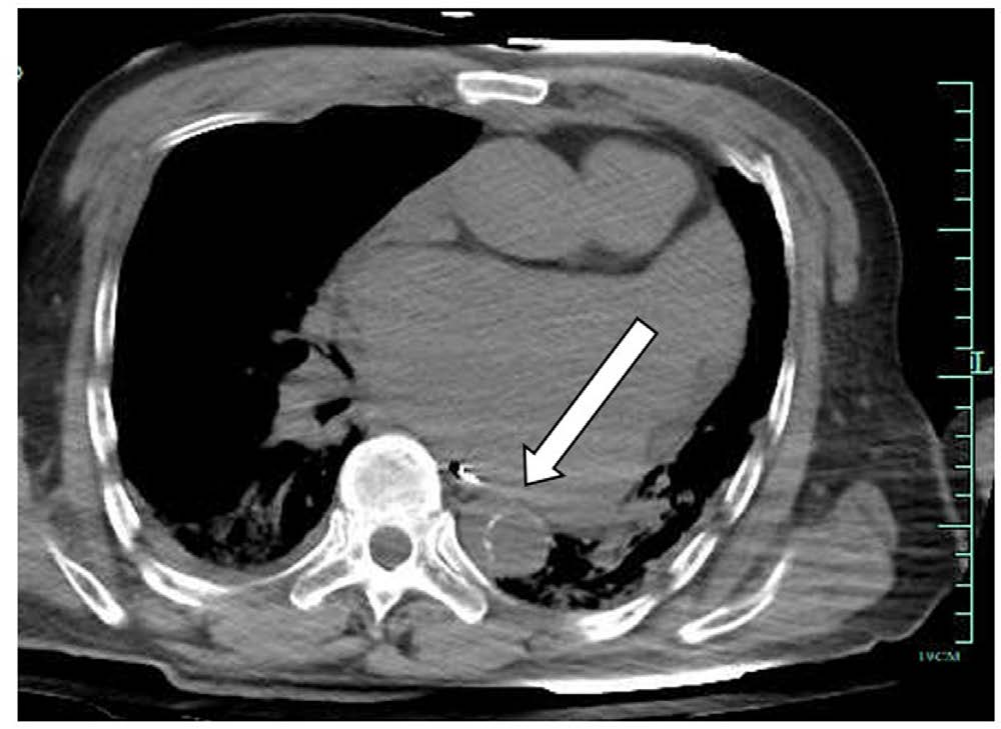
Computed tomography of the chest demonstrating displacement of the aorta calcification.
